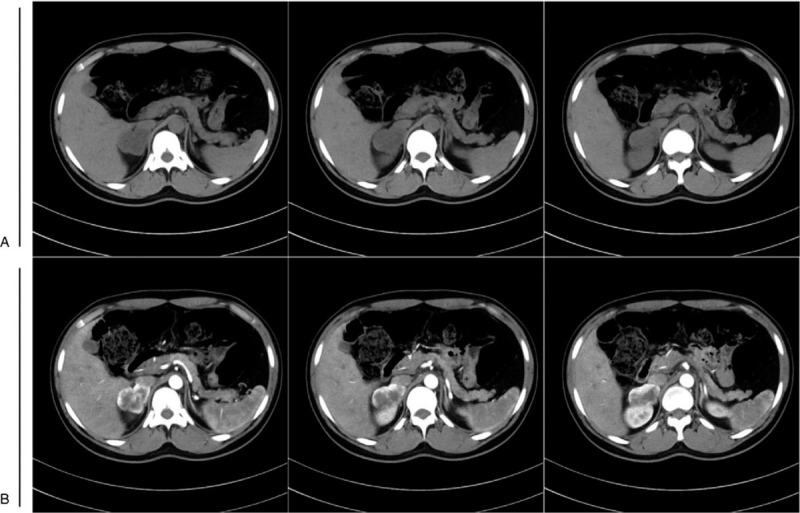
Computed tomography (CT) scan of the right adrenal mass. (A) The mass was showed on non-contrast CT scan. (B) The mass was enhanced in varying degrees on contrast CT scan.
